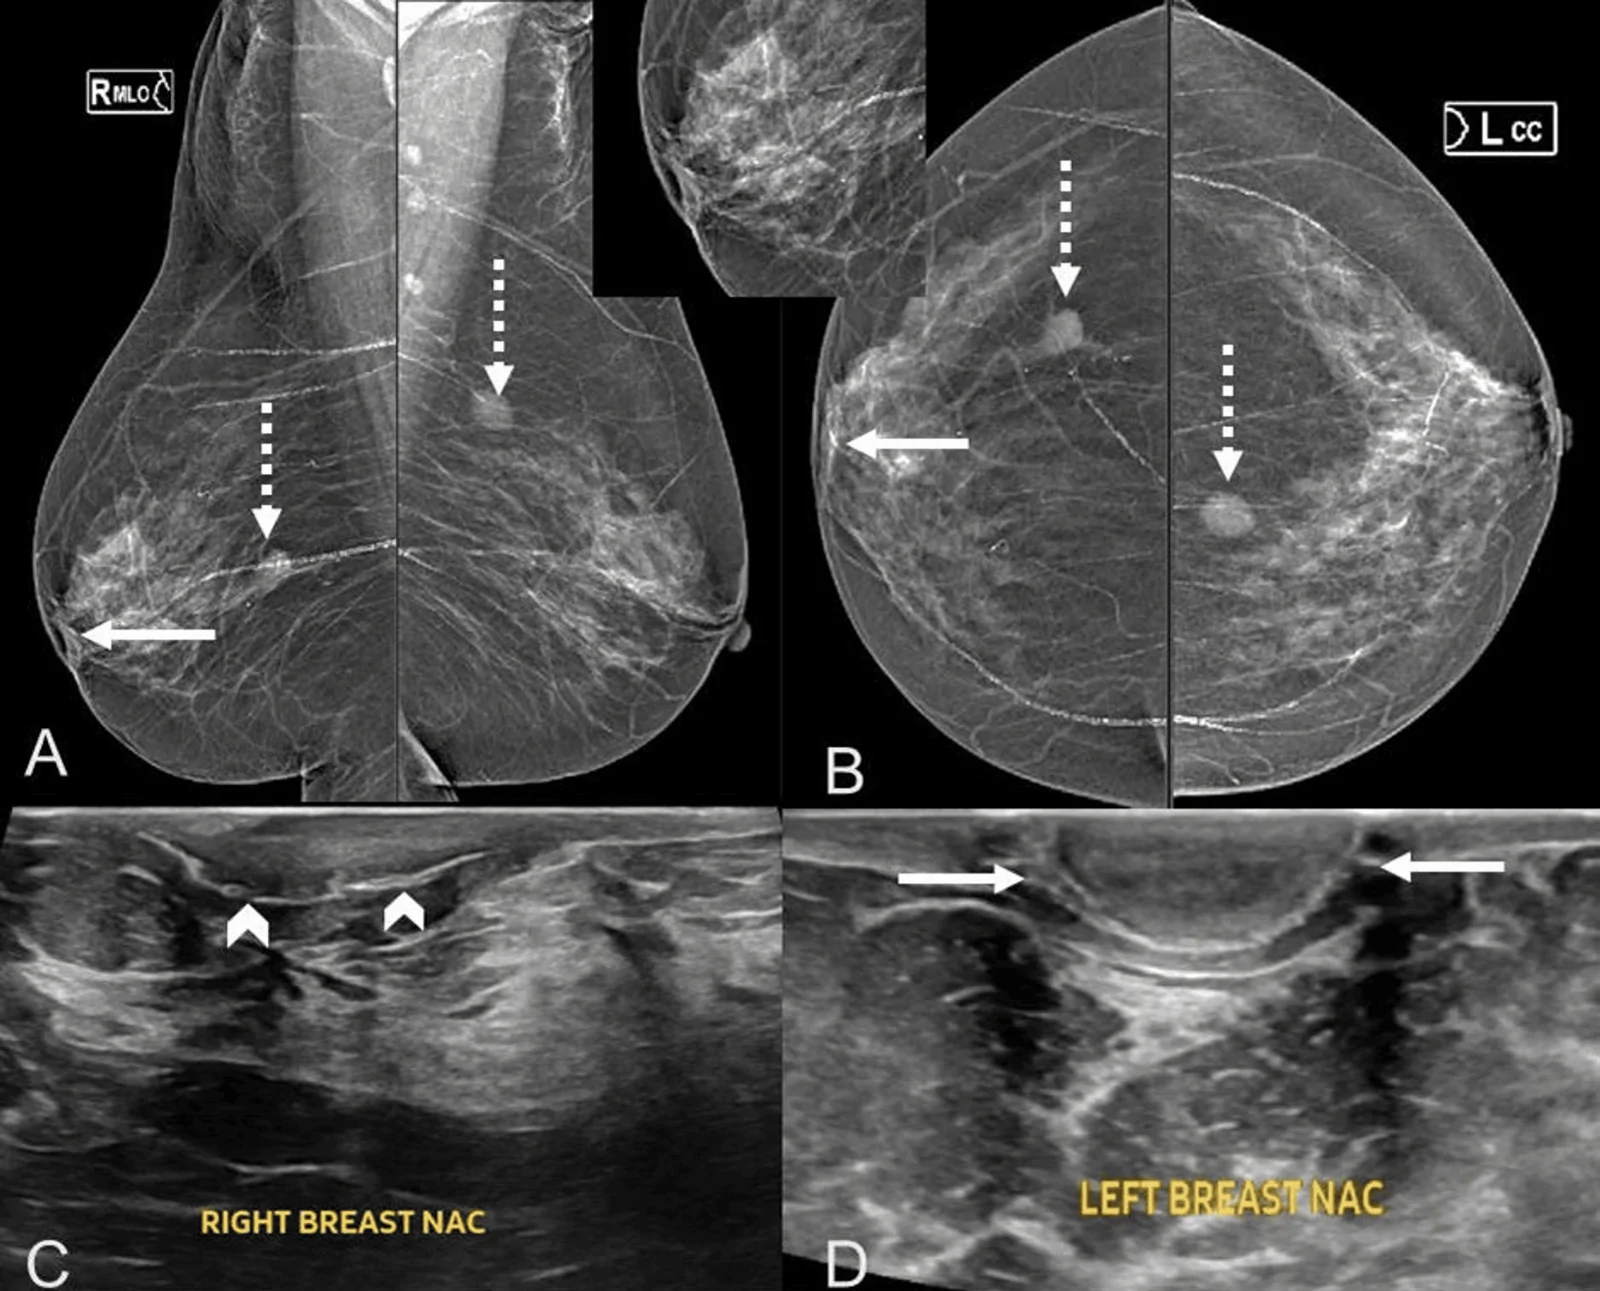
Mammographic and ultrasonographic findings demonstrating right nipple retraction with comparison to the normal contralateral nipple-areola complex (A and B) Mammogram showing retraction of the right nipple (solid white arrow), also showing a round, circumscribed, equal-density nodule in each breast (dotted white arrow). (C) Ultrasonogram representing retraction of the right nipple (arrowhead). (D) Normal left nipple-areolar complex (solid white arrows)
